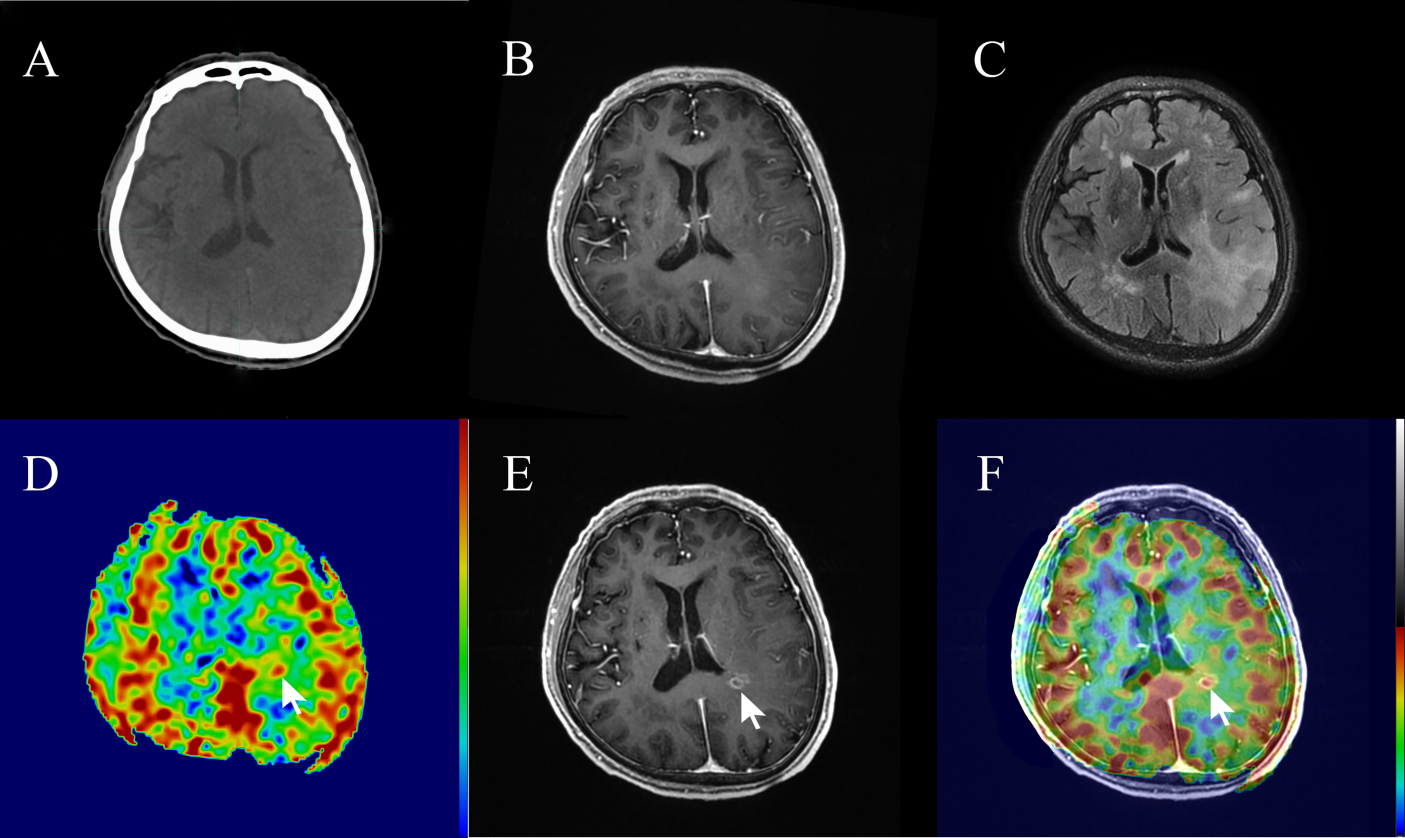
A 74-year-old patient with non-enhancing low-grade glioma (WHO grade II astrocytoma) showed focal contrast enhancement within a prior non-enhanced and high-perfusion area at 20 sessions of radiotherapy (40 Gy). (A) Planning plain-CT image. (B) Contrast-enhanced T1-weighted imaging (CE-T1WI) before radiotherapy. (C) T2 fluid-attenuated inversion recovery (T2 Flair) image before radiotherapy. (D) Three*-*dimensional arterial spin labeling (3D-ASL) image before radiotherapy. (E) CE-T1WI at 20 sessions of radiotherapy. (F) Fusion image of (D, E).
